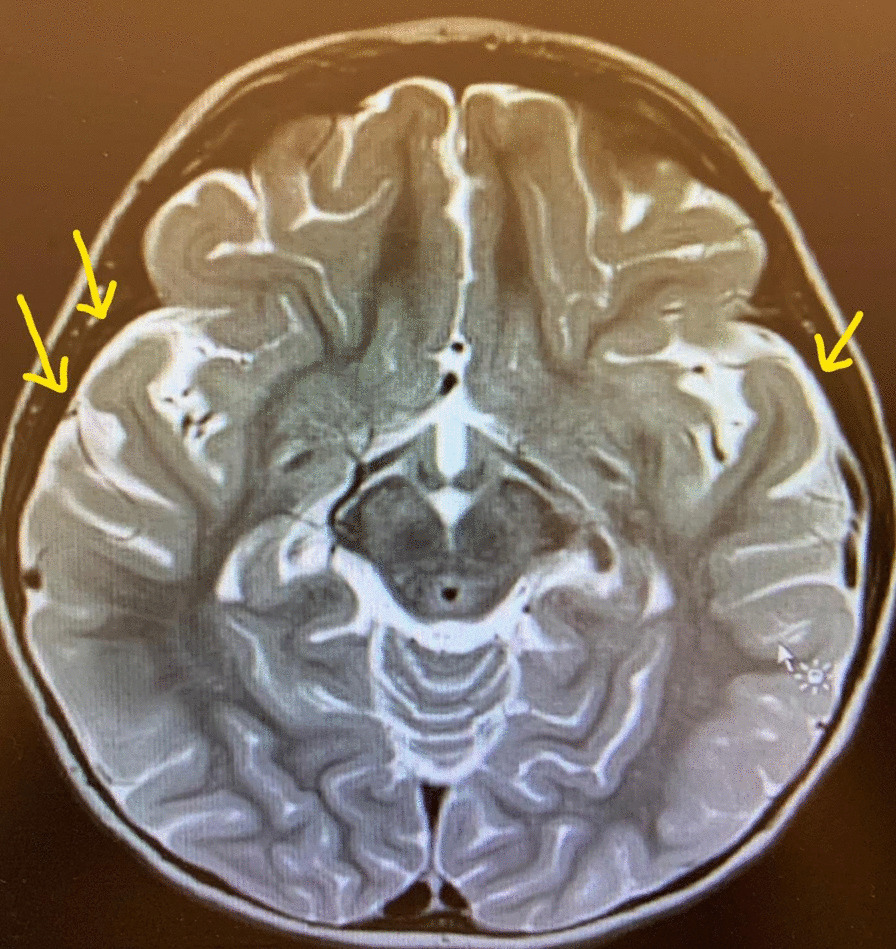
Magnetic resonance imaging (MRI) head - arrows pointing towards resolution of pockets of pus
